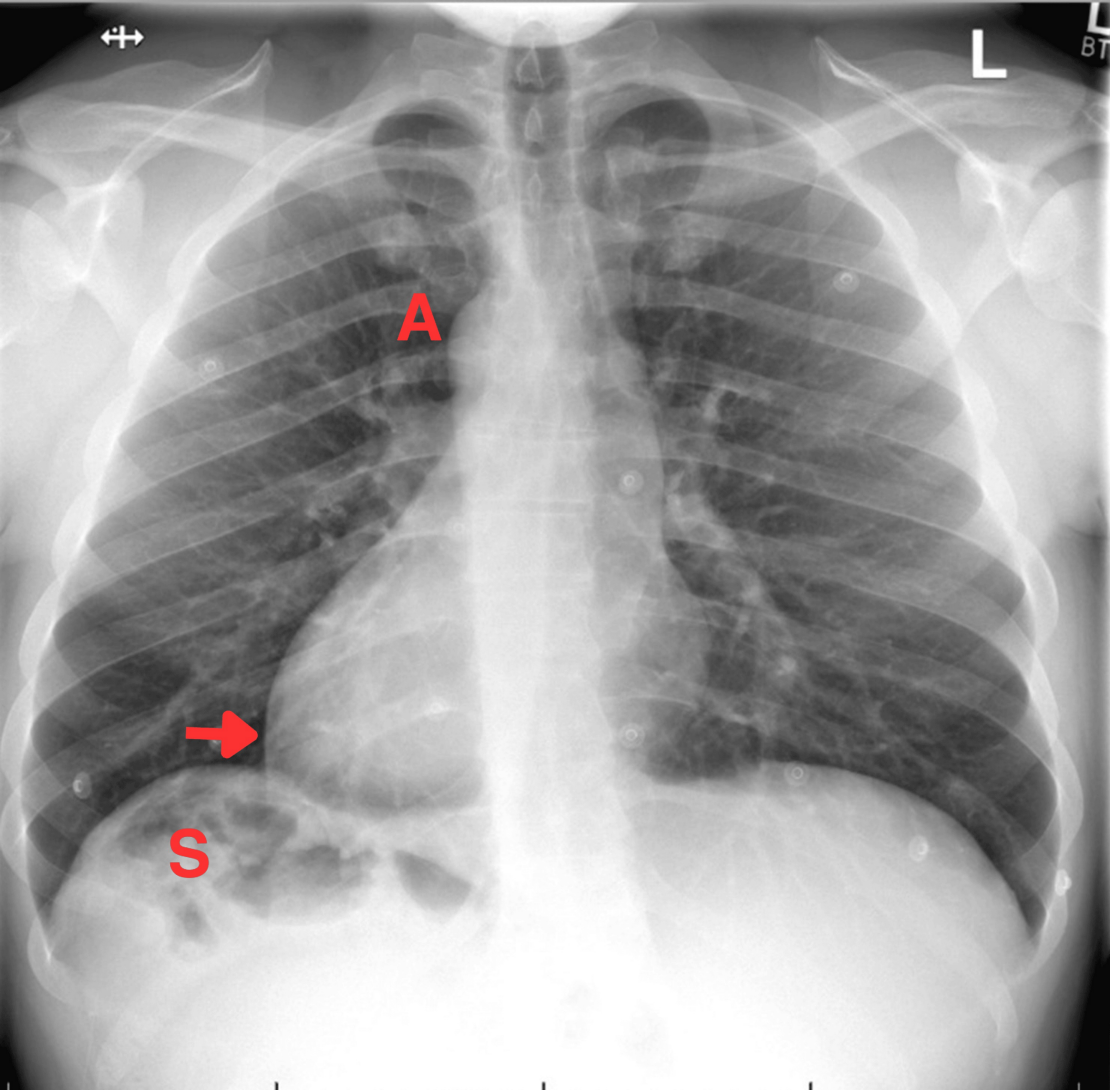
Chest Radiograph Demonstrating Situs Inversus Totalis An anteroposterior (AP) chest radiograph demonstrating dextrocardia, with the cardiac apex (arrow) and aortic arch (A) positioned on the right side of the thorax. A gastric air bubble (S) is visible beneath the right hemidiaphragm, confirming right-sided stomach orientation. These findings are diagnostic of situs inversus totalis (SIT).
